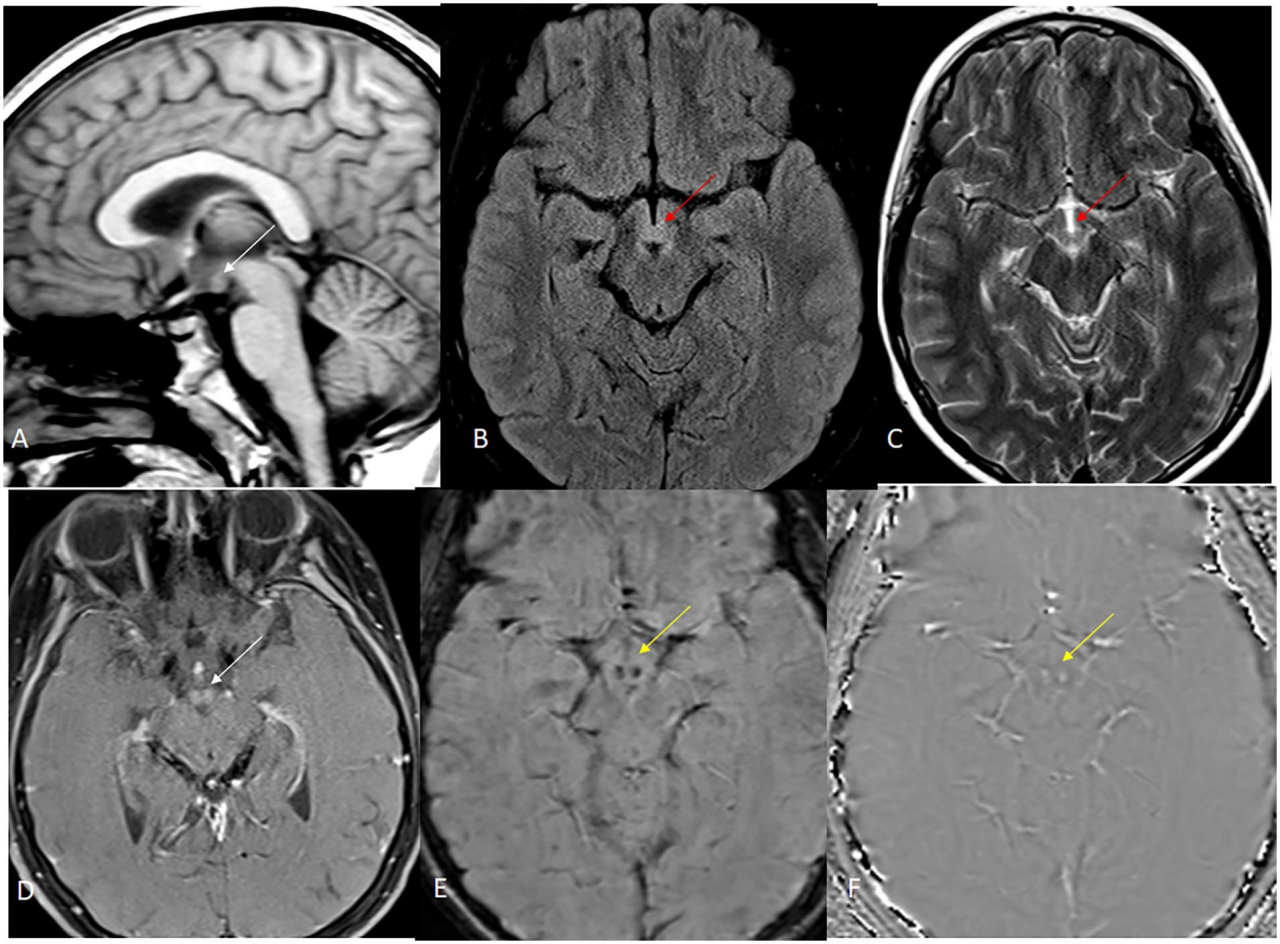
Repeat MRI performed 2 days later in our hospital demonstrates mild swelling of the mammillary bodies without T1 hyperintensity (image A, white arrow) with abnormal T2/FLAIR hyperintensity (images B and C; red arrows). Post-contrast image demonstrates mild enhancement (image D, white arrow). SWI/phase images show hemorrhage bilaterally (images E and F; yellow arrows).
